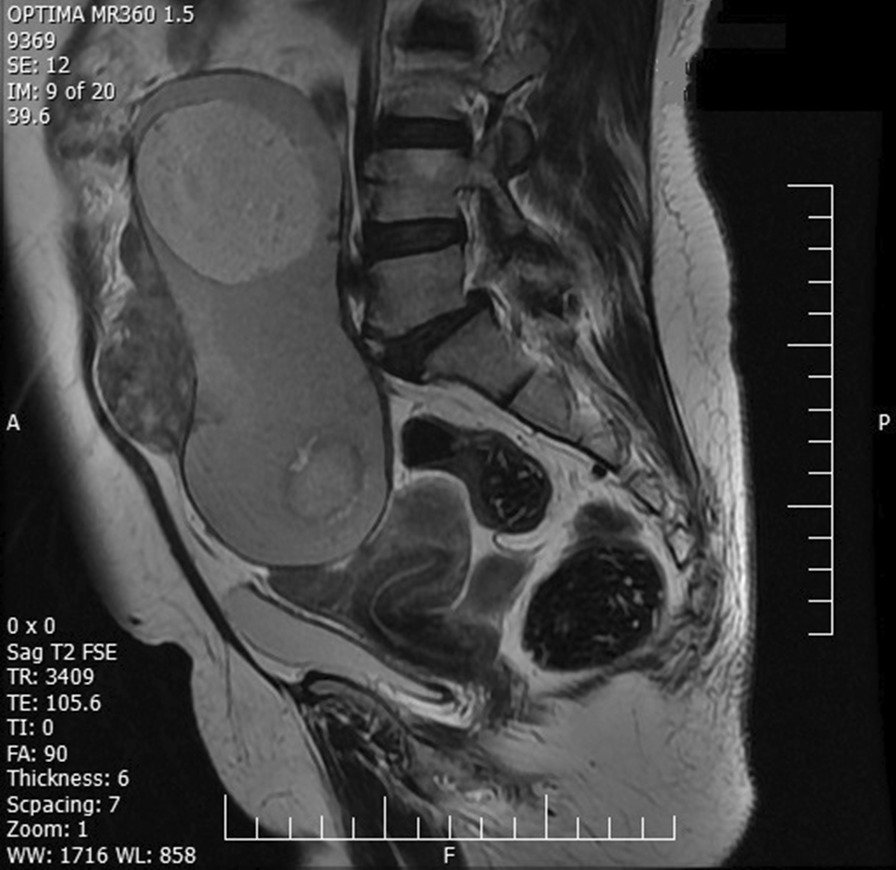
Sagittal T2-weighted fast spin echo magnetic resonance imaging image showing an intra-abdominal large solid-cystic mass, with fat foci and omental thickening in the vicinity of the tumor
